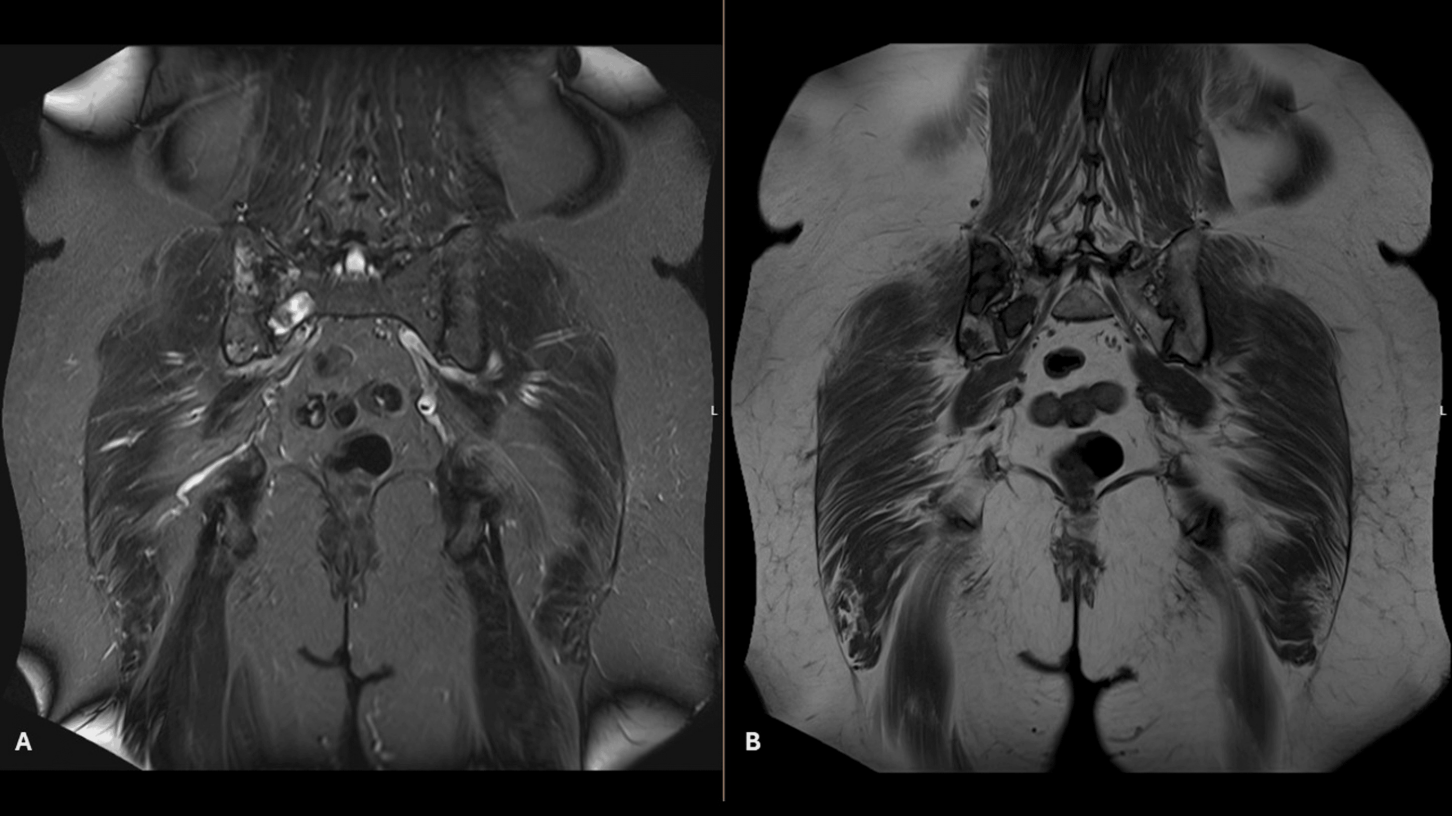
MRI of fibrous dysplasia lesions. Fibrous dysplasia lesions in the right sacral ala and adjacent right ilium. Coronal T2-weighted image on the left (A) and T1-weighted image on the right (B) showing hyperintense and hypointense signals, respectively.
